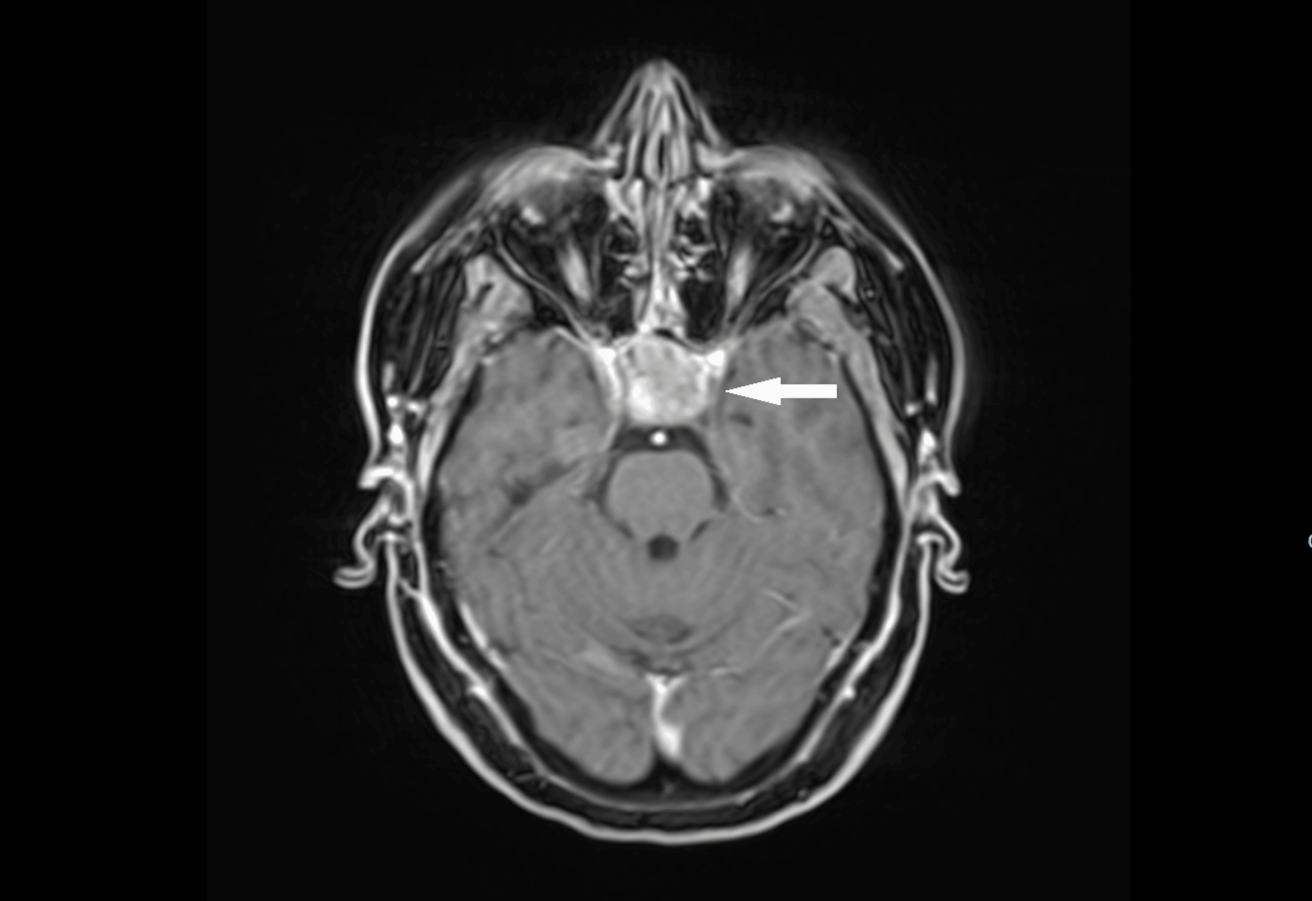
MRI of the pituitary gland, with the arrow indicating the macroadenoma of the pituitary gland
